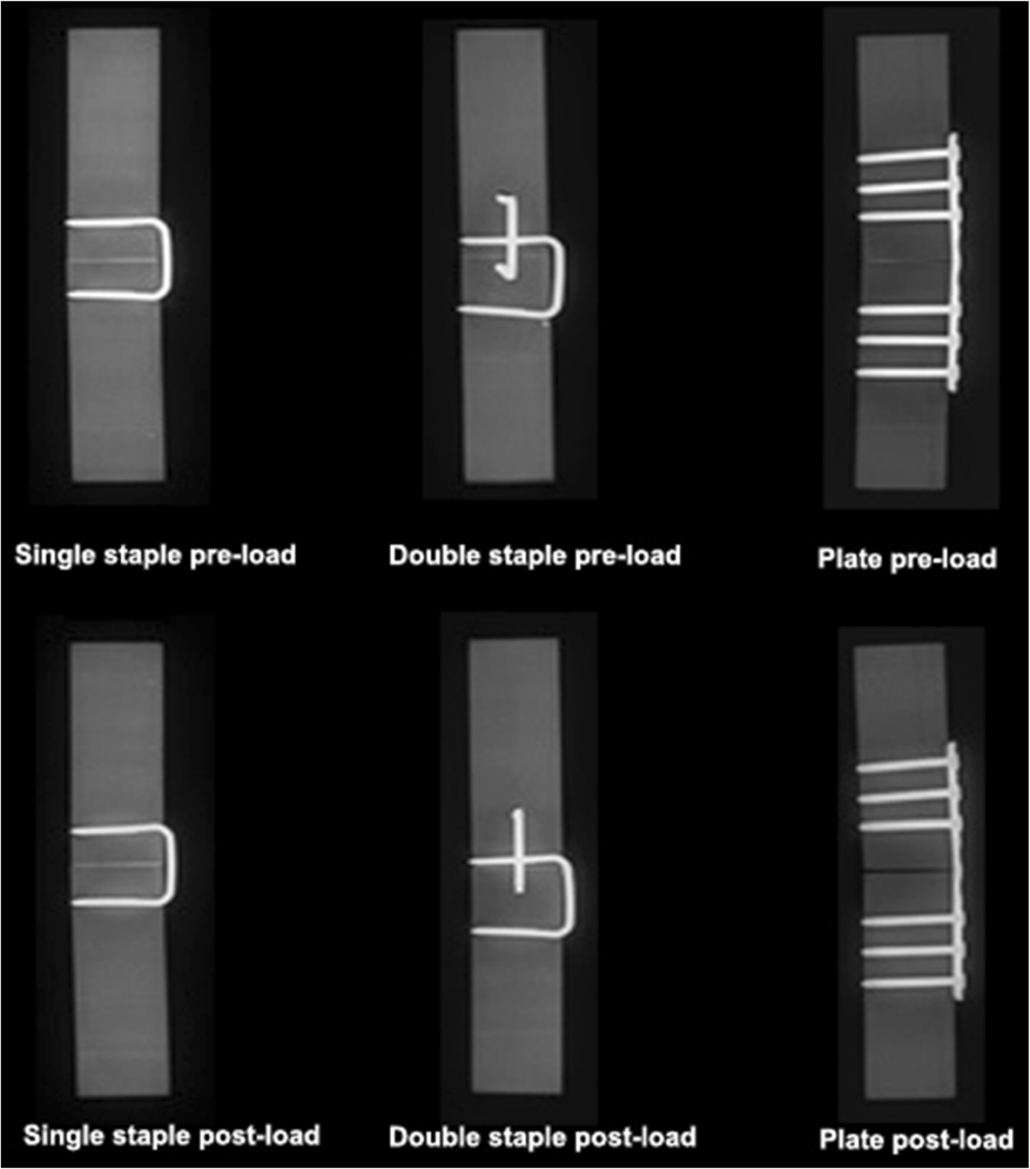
Examples of lateral radiographs taken of the constructs pre and post-loading. Subjective assessment shows consistent gap formation of plate constructs after loading compared to minimal to no gap formation in staple constructs after loading
